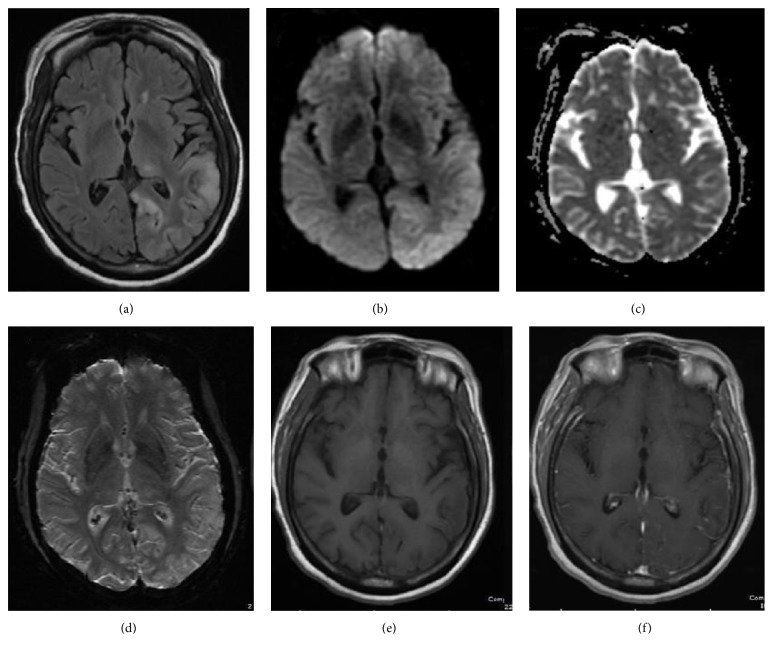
64-year-old female with confusion, right lower extremity weakness, and questionable encephalitis. Axial FLAIR MR image (a) shows increased signal intensity and gyriform swelling in the cortex and subcortical white matter of the left parietooccipital lobe and a focus in the left thalamus. Diffusion weighed (b) and attenuation diffusion coefficient, ADC, MR images (c) reveal mild decreased diffusivity. No susceptibility artifact or contrast enhancement is observed on axial susceptibility weighted image, SWI (d), and axial T1 weighted (e) and axial T1 weighted postgadolinium images (f), respectively.
